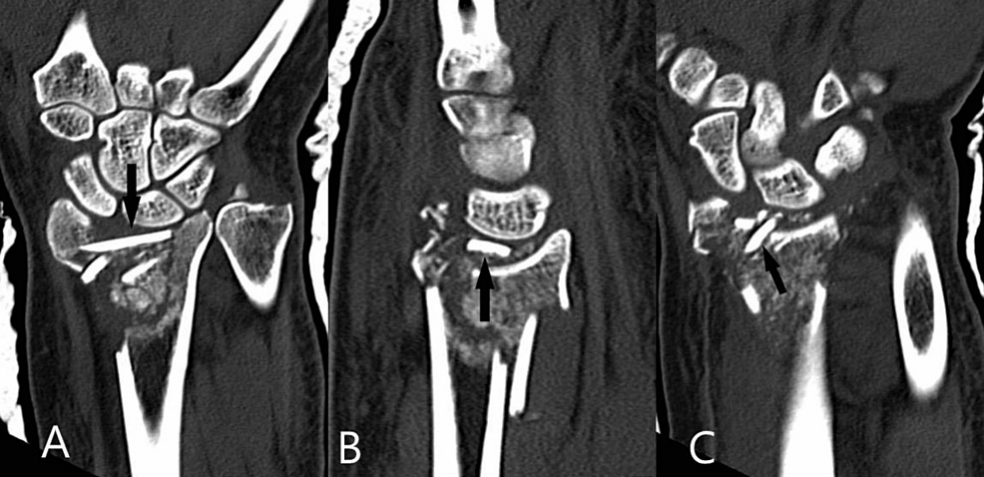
Computed tomography (CT) coronal and sagittal reconstructions A: This image demonstrates the displacement of cortical fragments within the radiocarpal joint, as indicated by the black arrow. These fragments were devoid of any soft tissue attachments. They were retrieved and reduced to the remaining dorsal distal radius. They were then stabilised with several suture knots. B: The black arrow demonstrates the displacement of the cortical fragments within the radiocarpal joint. The black arrow demonstrates the intra-articular position of the metaphyseal fragments.
